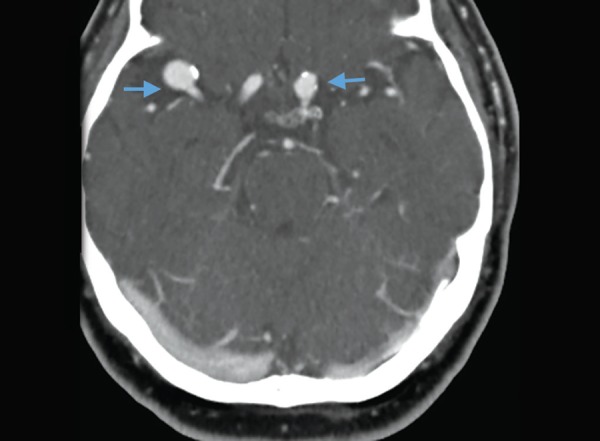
Aneurysms on CT angiography: two contrast filled out pouchings (arrows) along right MCA and left supraclinoid ICA
